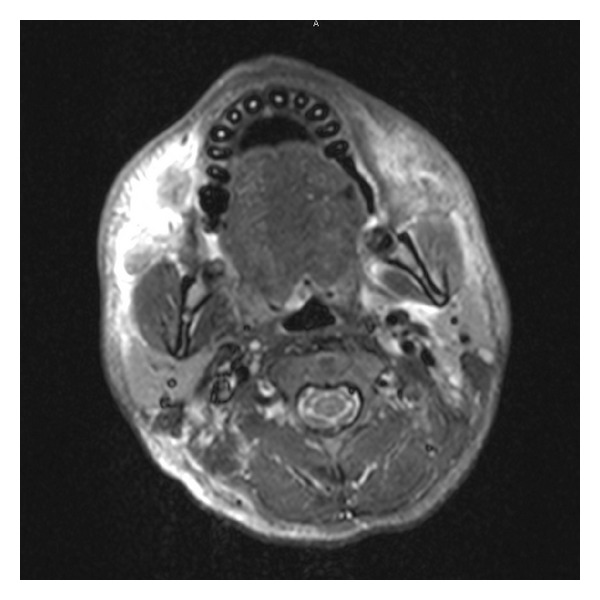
CT maxillofacial scan demonstrating extensive inflammatory changes of the left face without evidence of abscess. These changes were later determined to be nonspecific inflammatory changes resulting from ALCL-associated HPS.
